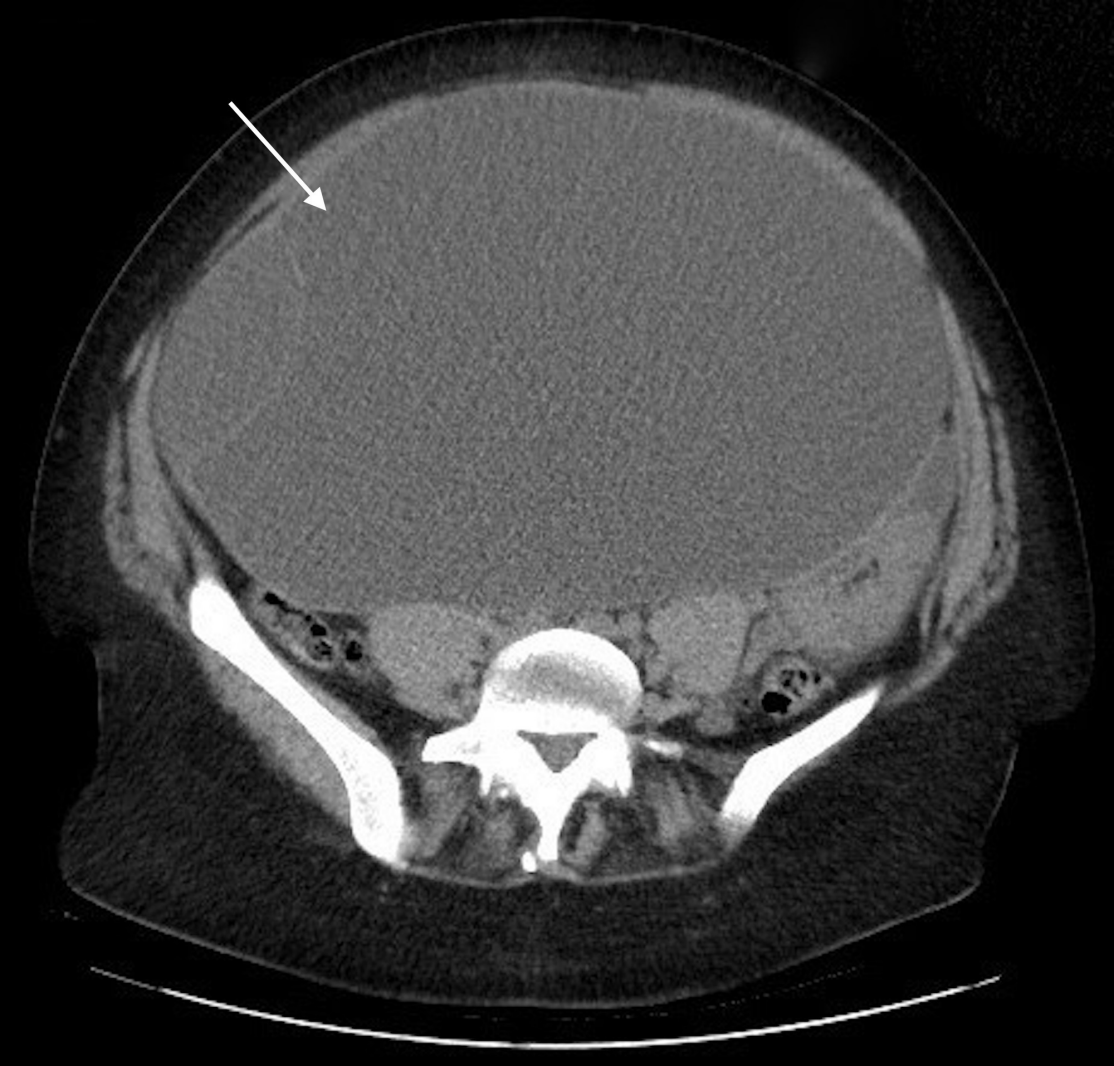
Axial computed tomography image of the abdomen at the level of the iliac crest showing a large cystic mass measuring up to 30 cm.
